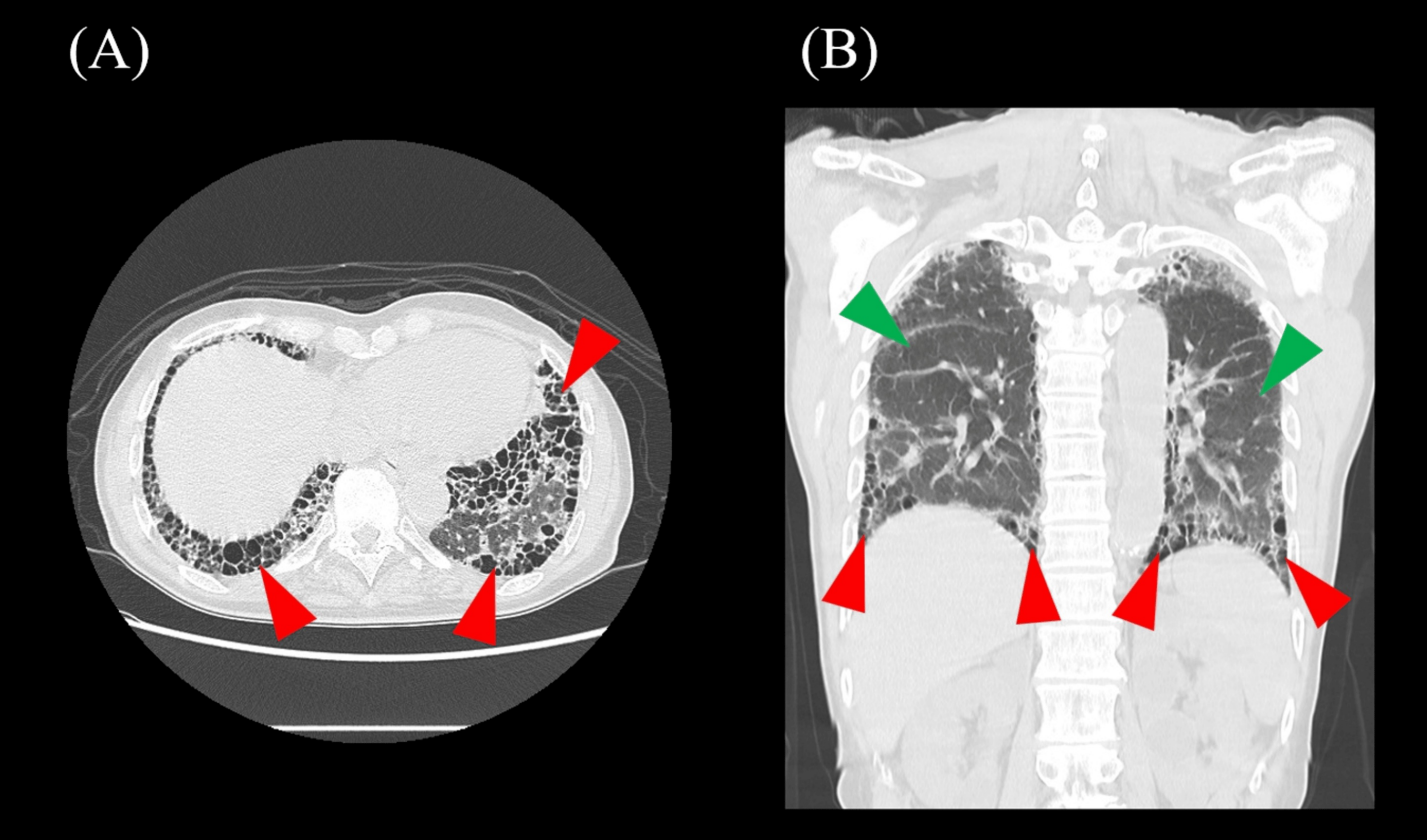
Results of the chest computed tomography (CT) two months after stroke. (A, B) Chest CT showing interstitial opacities predominantly distributed in the peripheral regions of both lungs, accompanied by honeycombing (red arrowheads), suggesting interstitial pneumonia with a usual interstitial pneumonia (UIP) pattern. Overall, decreased aeration and increased lung density are observed (green arrowheads), raising the possibility of acute exacerbation. (A) Axial section; (B) coronal section.
